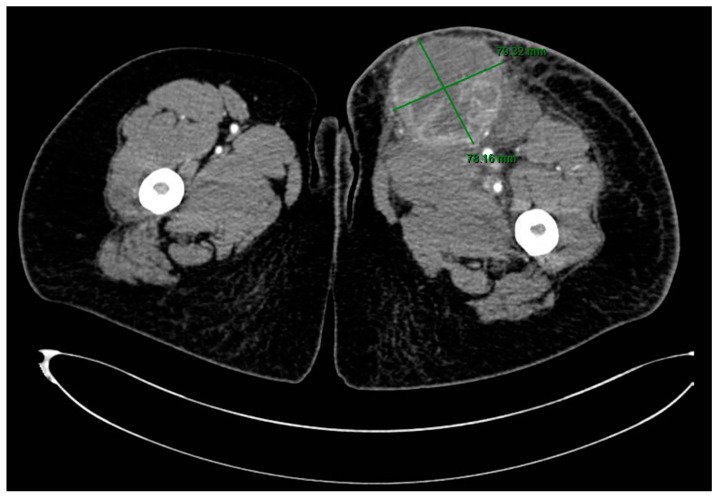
CT scan of the lower left limb showing a large mass in the upper thigh.
